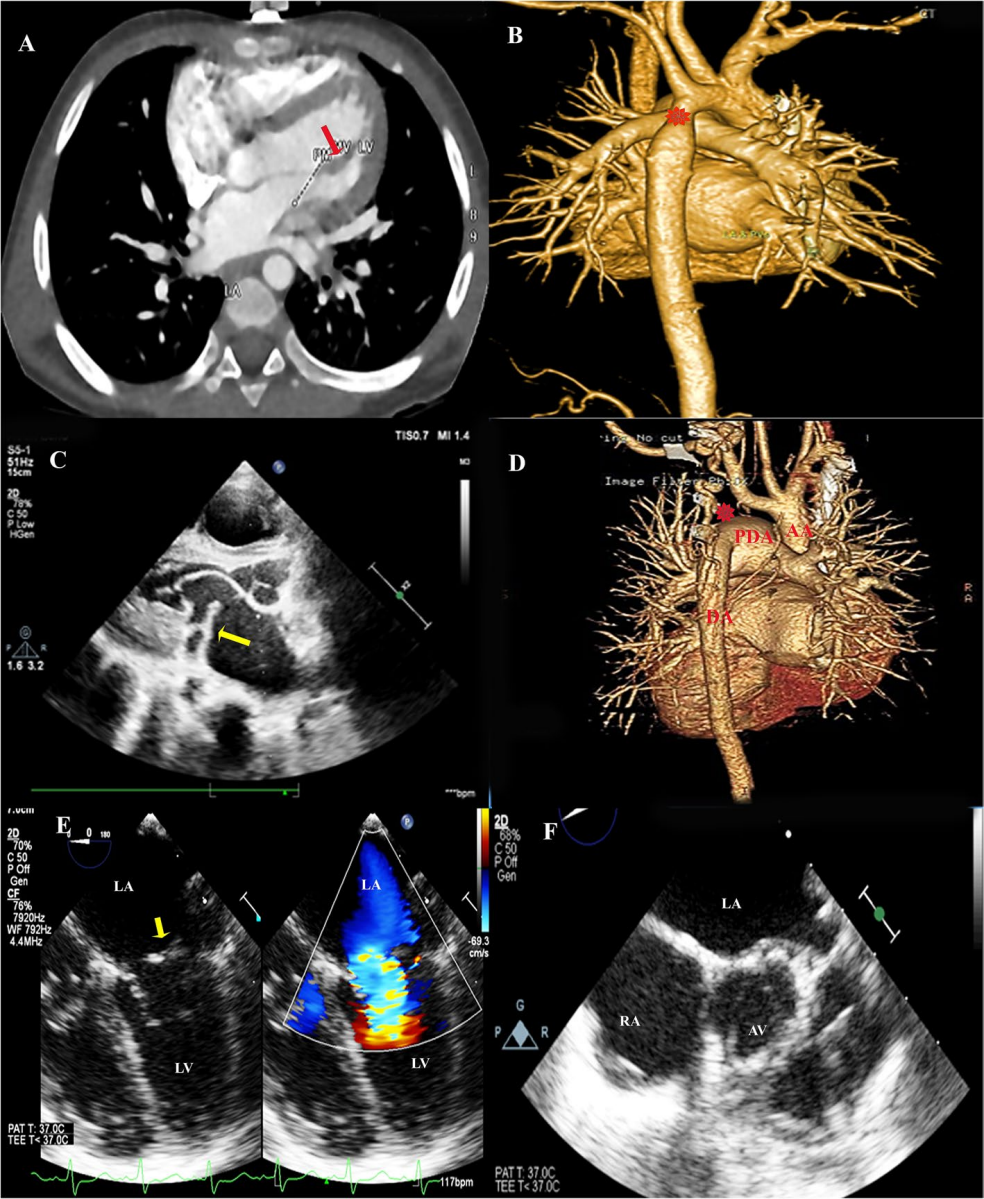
Examples of Diagnostic imaging for some of the SC cases requiring surgical intervention. A, B) axial CT cut showing dilated LV& LA with parachute mitral valve (red arrow) and 3D volume rendering demonstrating COA in the same patient (red asterisk). C, D) TTE of long parasternal view demonstrating supramitral membrane and 3D volume rendering CT showing interrupted aortic arch in the same patient (red asterisk at the interruption). E, F) TEE of four-chamber view demonstrating turbulent flow across supramitral membrane (yellow arrow) with parachute mitral and short axis view showing BAV in the F panel. AA: ascending aorta, AV: aortic valve, DA: descending aorta, LA: left atrium, LV: left ventricle, PDA: patent ductus arteriosus, and RA: right atrium
